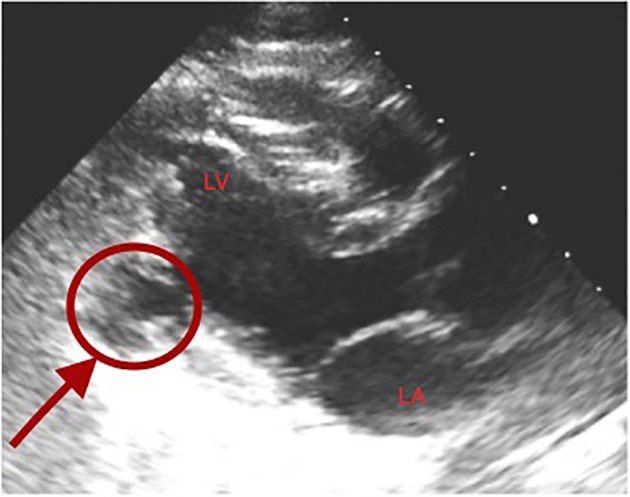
Transthoracic echocardiogram demonstrating left ventricular pseudoaneurysm (arrow) in the distal inferolateral wall.
